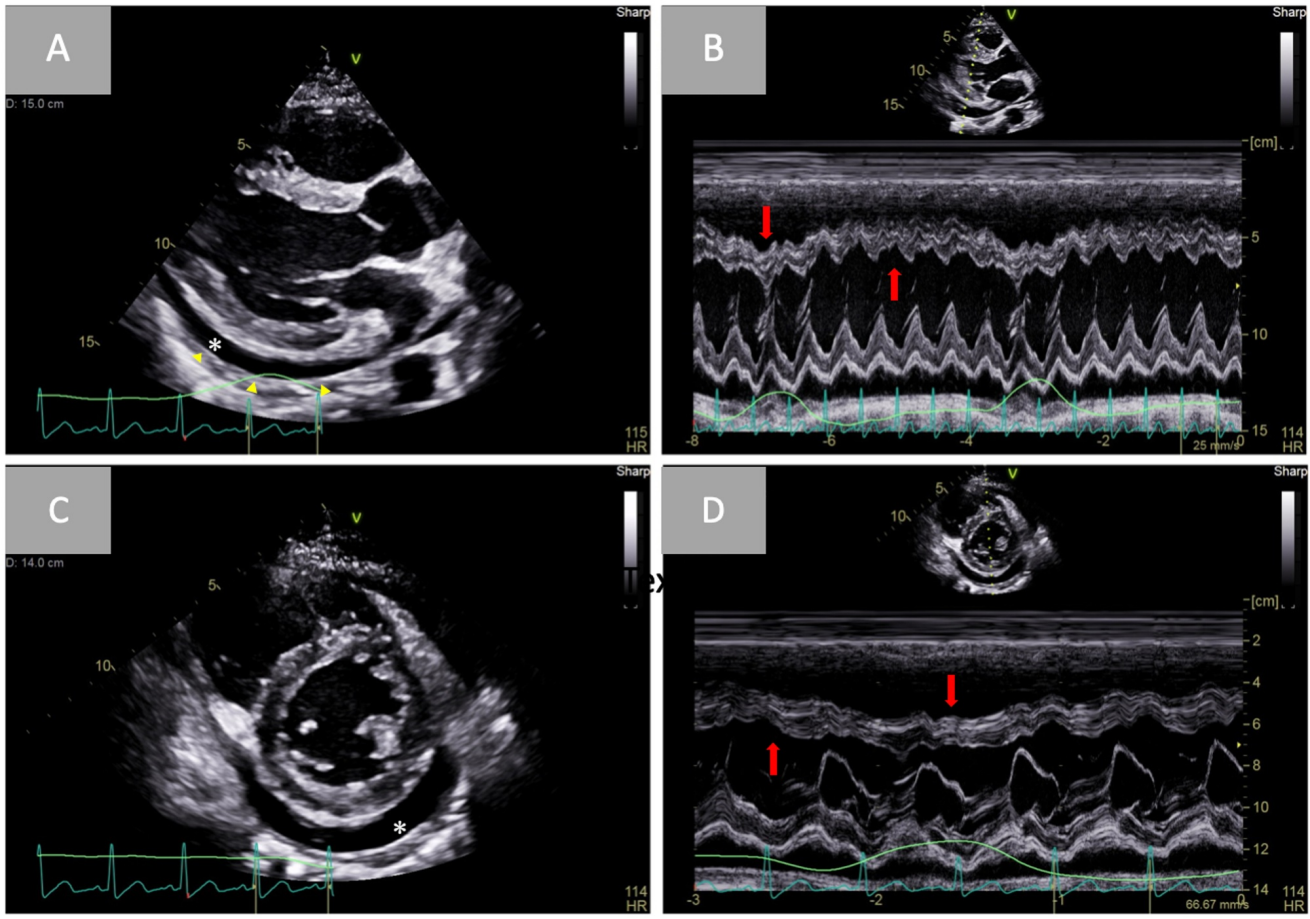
Demonstration of pericardial effusion with respirophasic interventricular septal shifting Two-dimensional parasternal long-axis (A) demonstrating increased pericardial thickness (yellow arrowheads) and a moderate pericardial effusion (white asterisk) following pericardiocentesis. M-mode echocardiography (B) depicting ventricular septal shift towards the left ventricle (downward arrow) during inspiration and towards the right ventricle (upward arrow) during expiration. Simultaneous respirometric recording is shown at the bottom of the figures (green line). Two-dimensional parasternal short axis (C) showing a moderate circumferential pericardial effusion (white asterisk). M-mode echocardiography (D) depicting ventricular septal shift towards the right ventricle (upward arrow) during expiration and towards the left ventricle (downward arrow) during inspiration.
